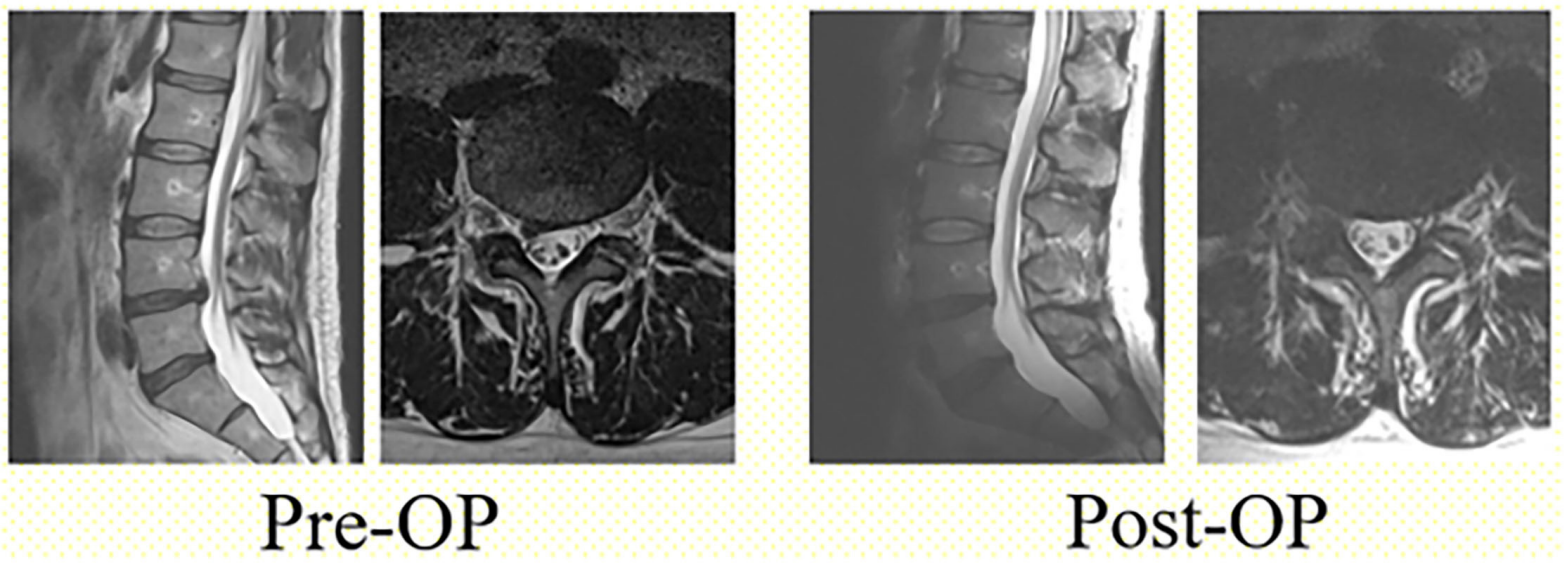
T2-weighted sagittal and axial views before and after surgery.
